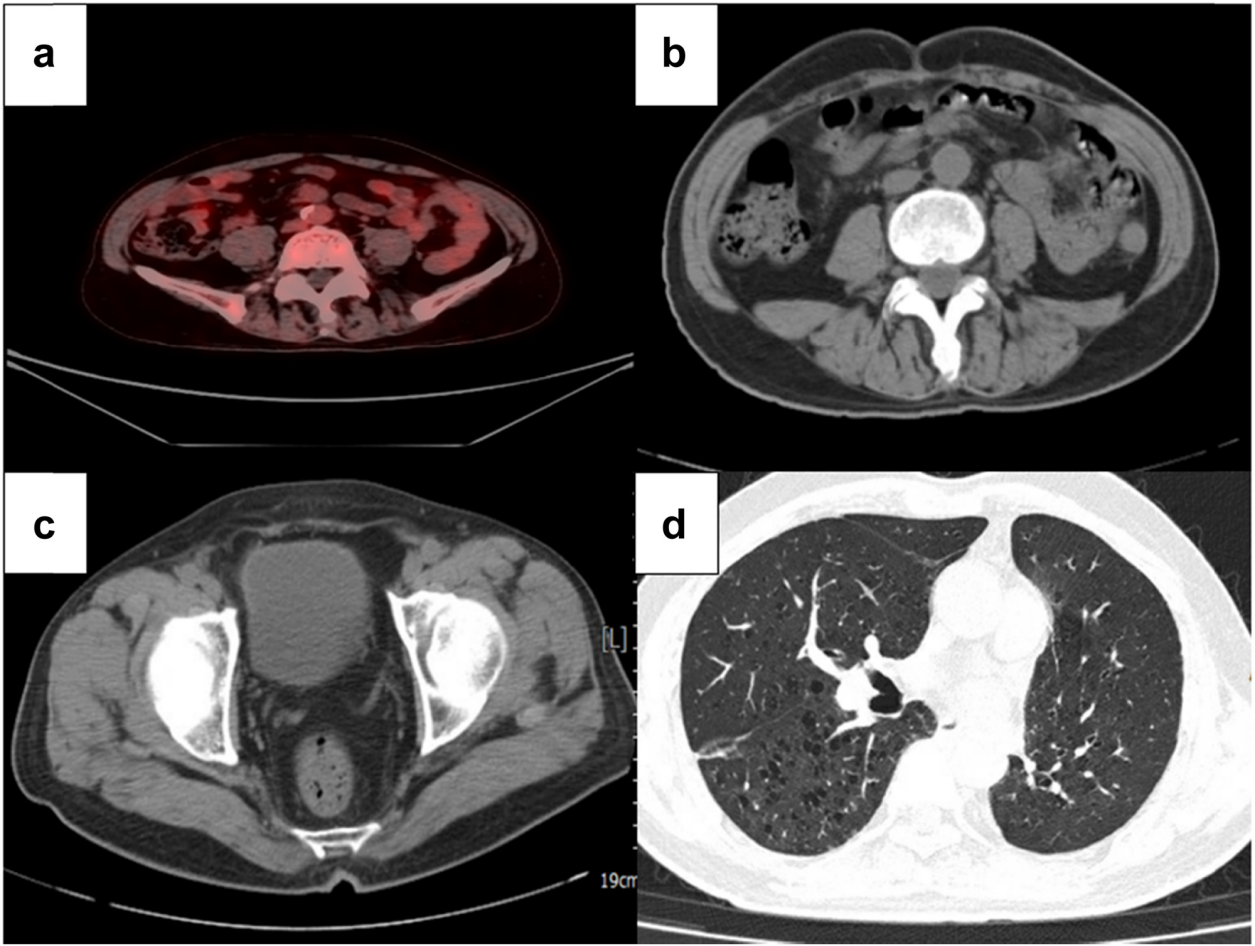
Follow-up CT scan and PET–CT scan showed no signs of disease relapse. (a) PET–CT scan performed at 1 month after standard chemotherapy, showing complete remission of lymphoma. (b) CT scan performed at 3 months after standard chemotherapy, showing complete remission of lymphoma. (c) CT revealed no signs of relapse in the primary site 3 months after TURBT. (d) CT revealed no signs of relapse in the primary site 3 months after surgical resection of the left upper lobe.
